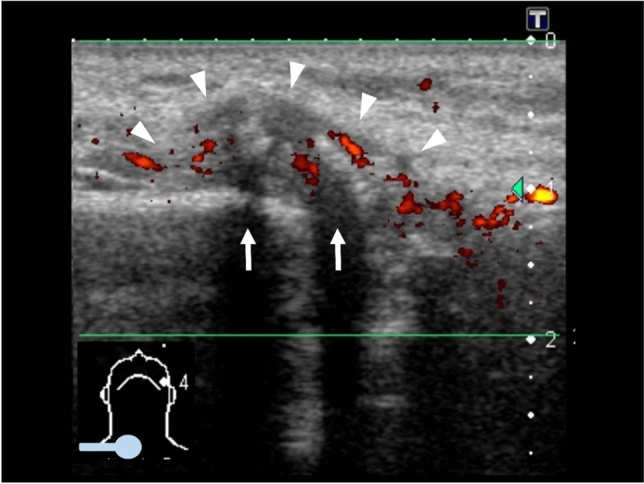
Power Doppler US on October 5, 2010 showed a hypoechoic solid mass (arrowheads) destroying the cortex of the clavicle (arrows), and with weak signals in the internal mass and surrounding blood flow
